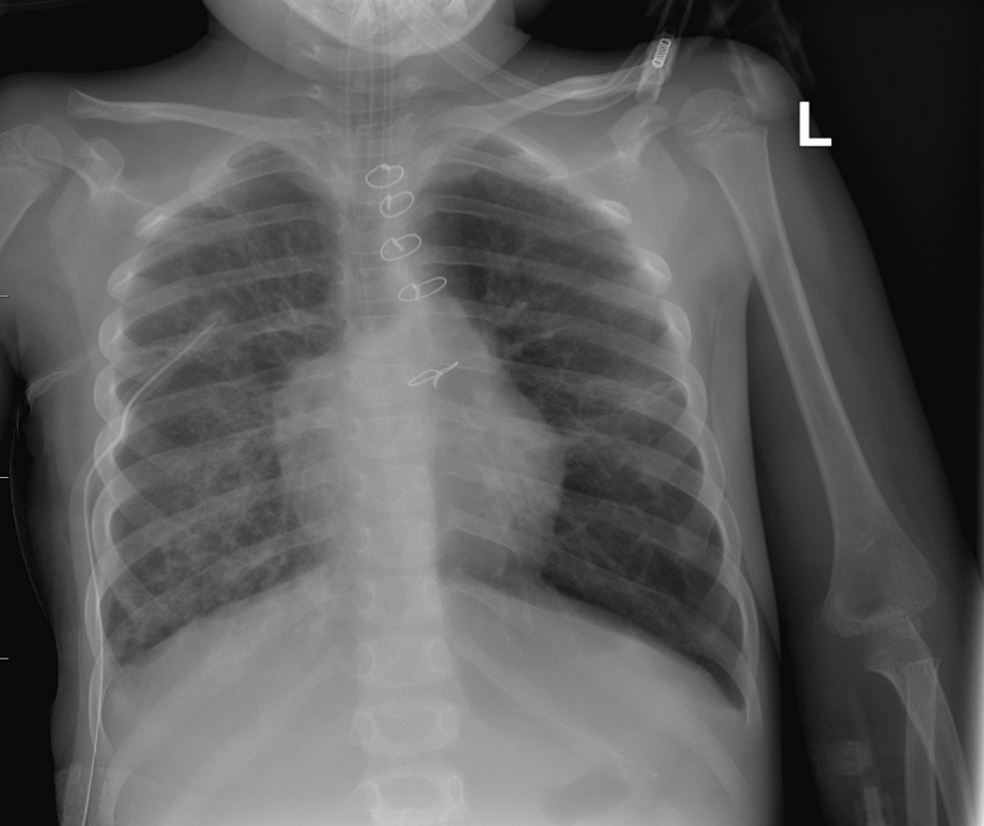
The frontal chest radiograph shows remarkable interval regression in the previously seen consolidation of the right hemithorax.
